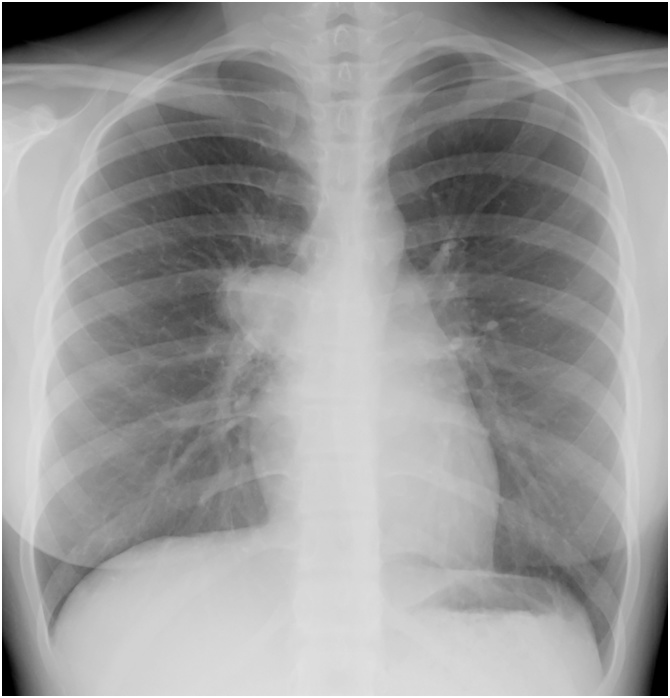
Chest plain radiography shows a 4.0 × 3.5 cm tumour adjacent to the mediastinum.
